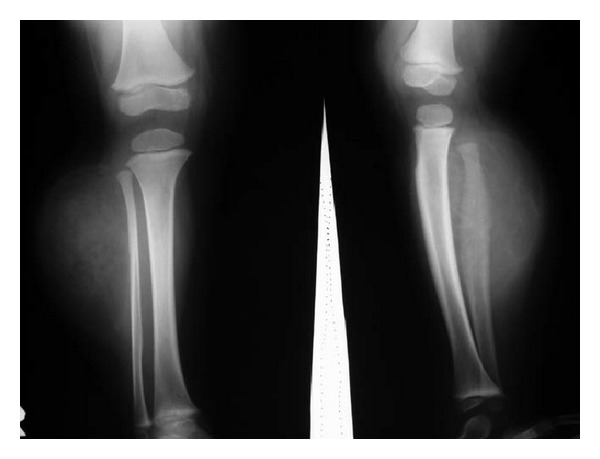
Plain radiograph of Case  2 showed a soft tissue mass with a more diffuse fatty-like radiolucent component. Although bowing deformity was not seen, periosteal thickening of the fibula was nicely depicted.
